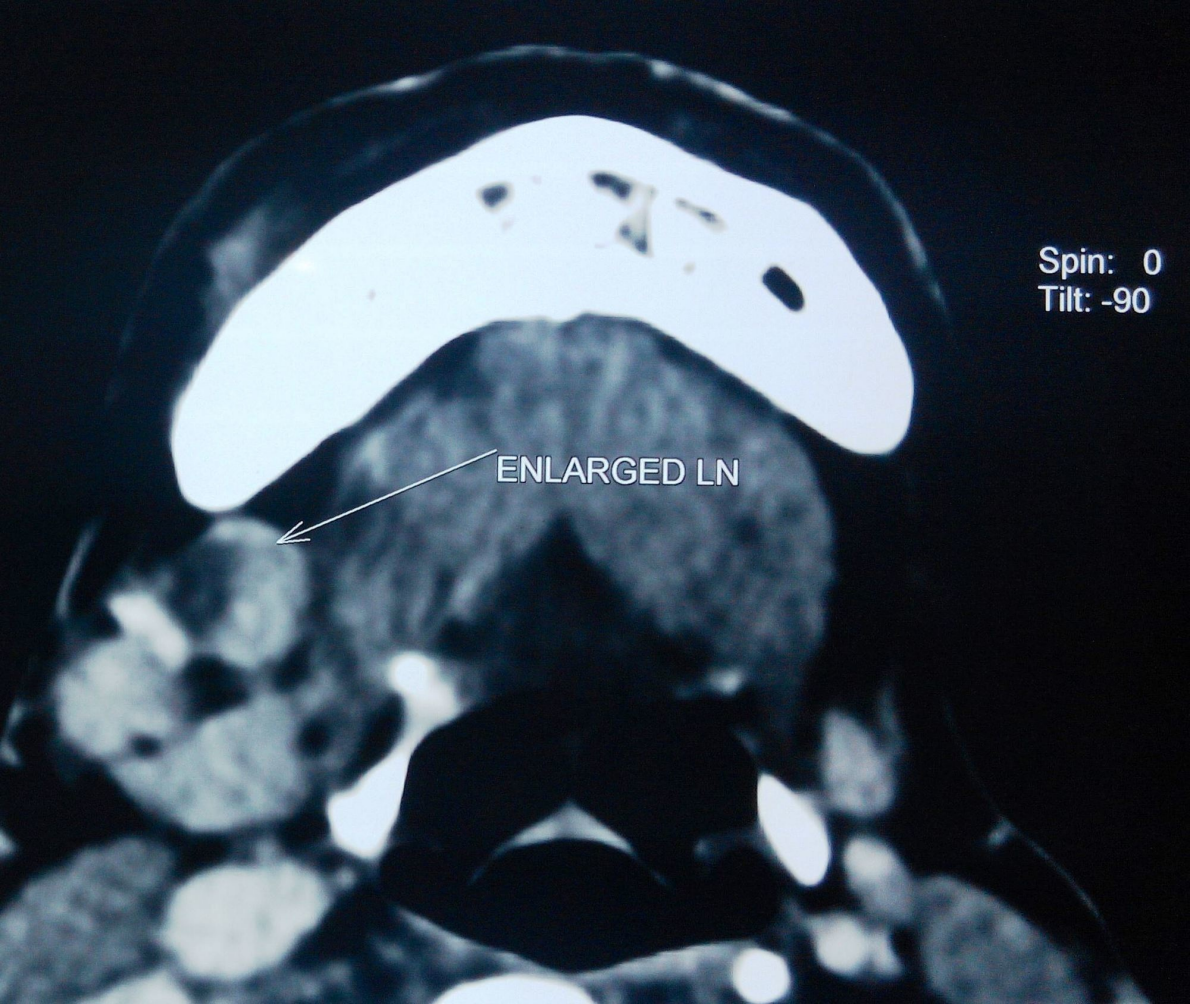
Axial section of contrast-enhanced computed tomographic image showing few prominent lymph nodes at level IA and IB of right side of neck (largest measuring 1.7 × 1.3 cm), one of the prominent lymph nodes showing necrosis within it.
